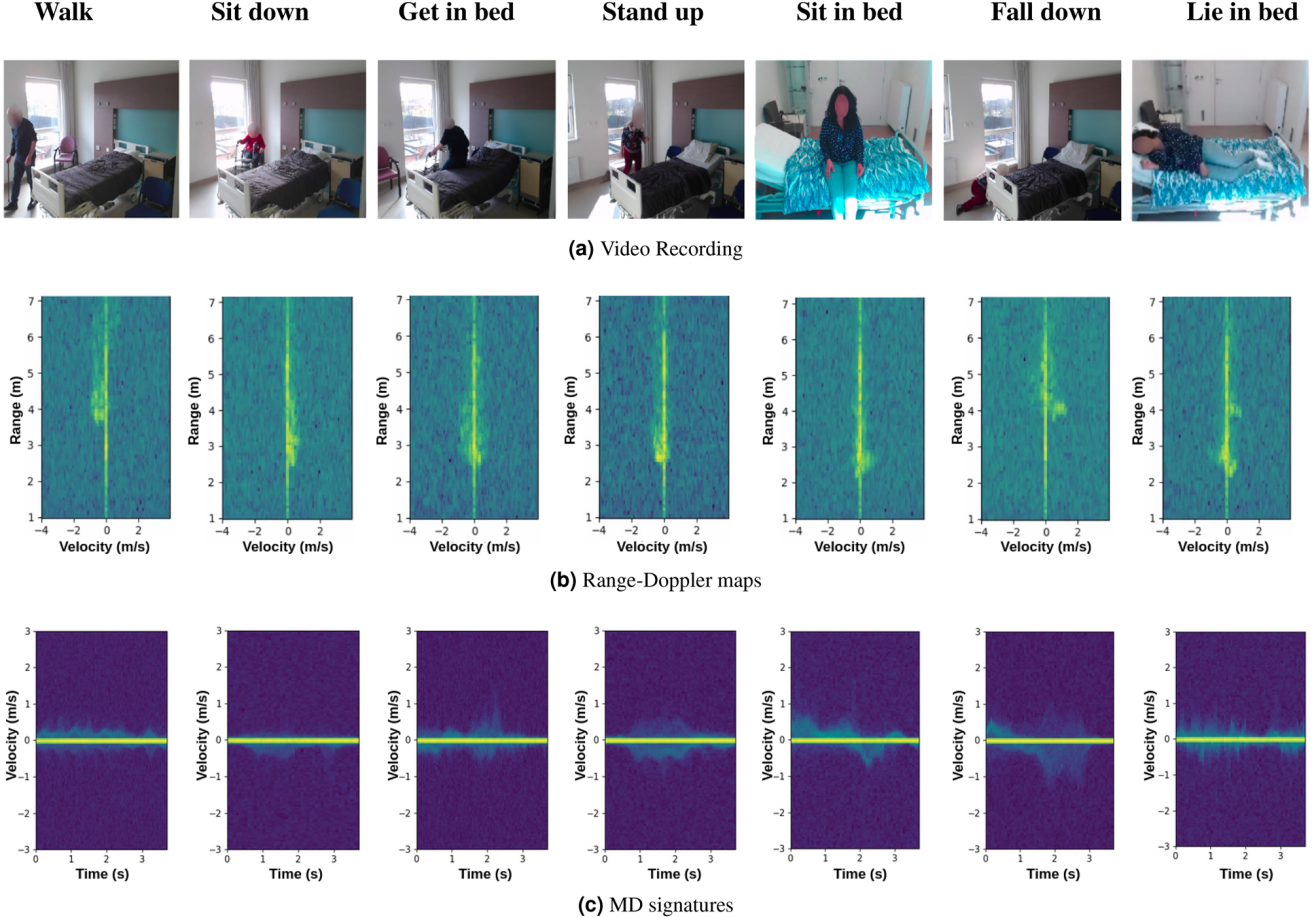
Visualisation of input data in different forms (adapted from Bhavanasi et al.^14^). In (a) example activities are seen, (b) shows the corresponding Range–Doppler maps in dB, and (c) shows the MD signatures in dB.
